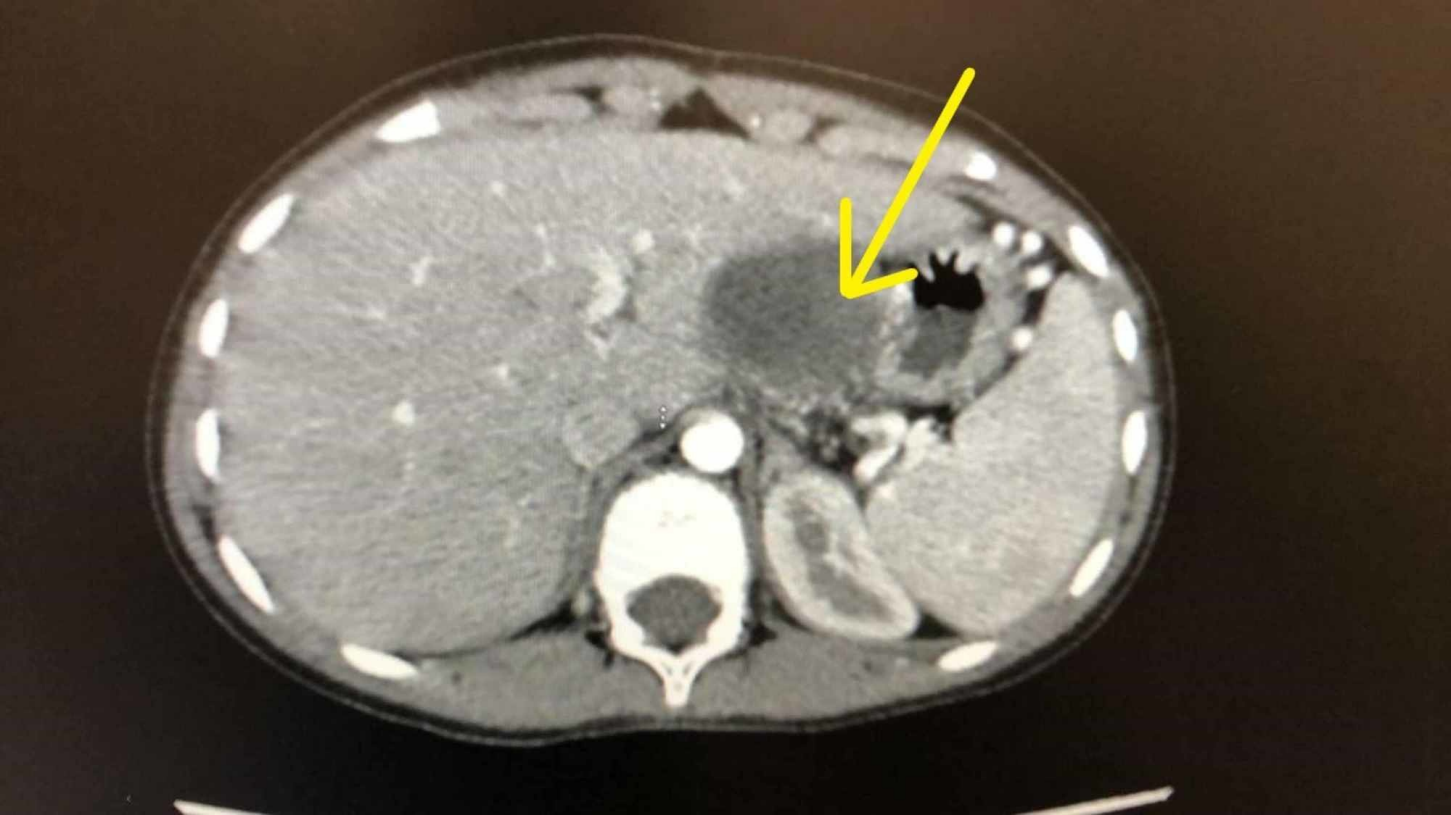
CT scan showing the lesion after chemotherapy, measuring 3.4 x 3.3 x 3.5 in diameter.
